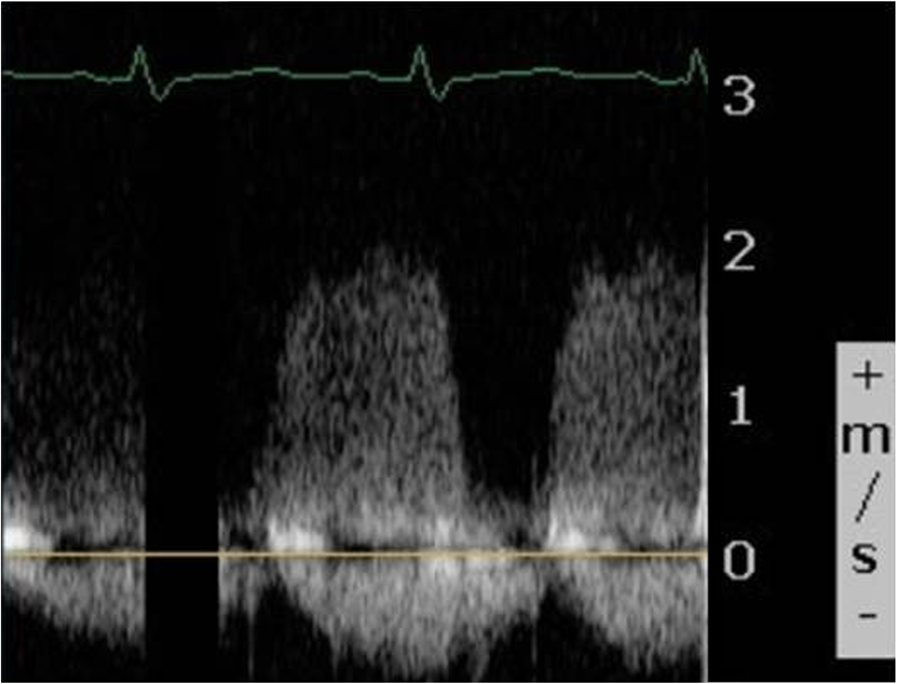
Continuous wave Doppler across the tricuspid valve showing increased inflow velocities.
